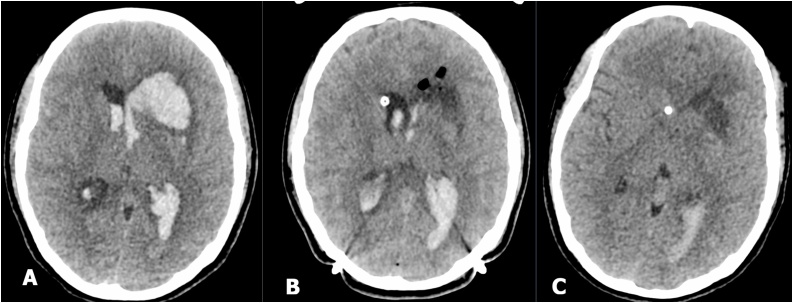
A. Head CT scan a few hour after onset, B. Head CT scan image after stereotactic aspiration immediately, and C. Head CT scan image taken a week after surgery.
